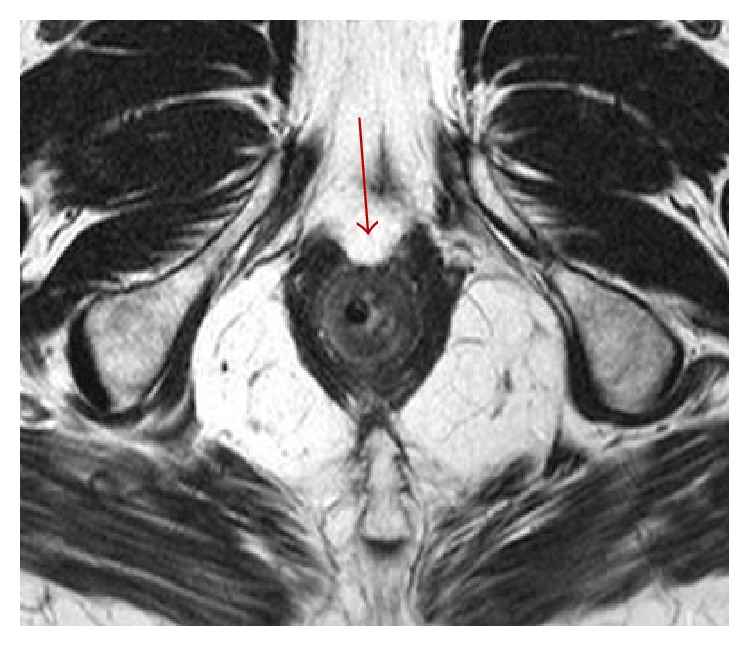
MR T2-weighted axial image of the anal sphincter complex taken perpendicular to the intra-anal marker: a focal defect (arrow) is visible from 11 to 1 (anal clock) after STARR procedure, responsible of episodes of fecal incontinence.
